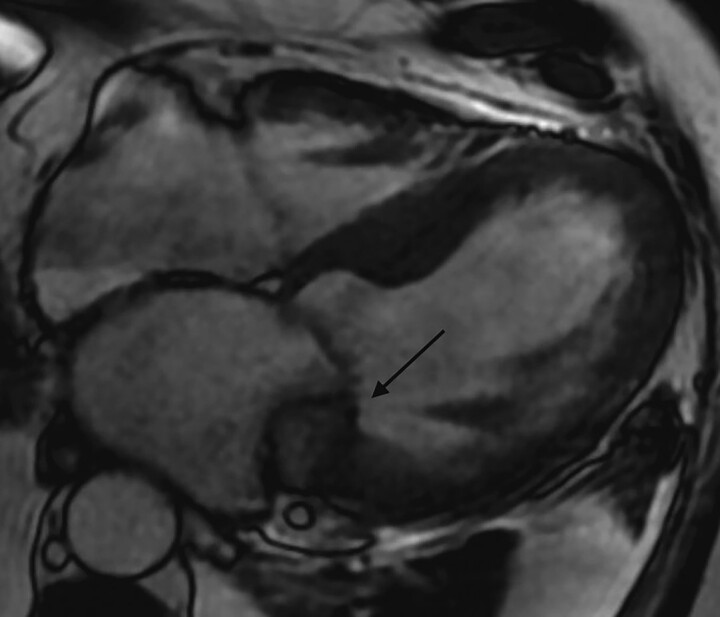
Cardiac magnetic resonance imaging. Late-gadolinium enhancement imaging showing hypointense mitral annulus mass with signal inhomogeneity (arrow).
